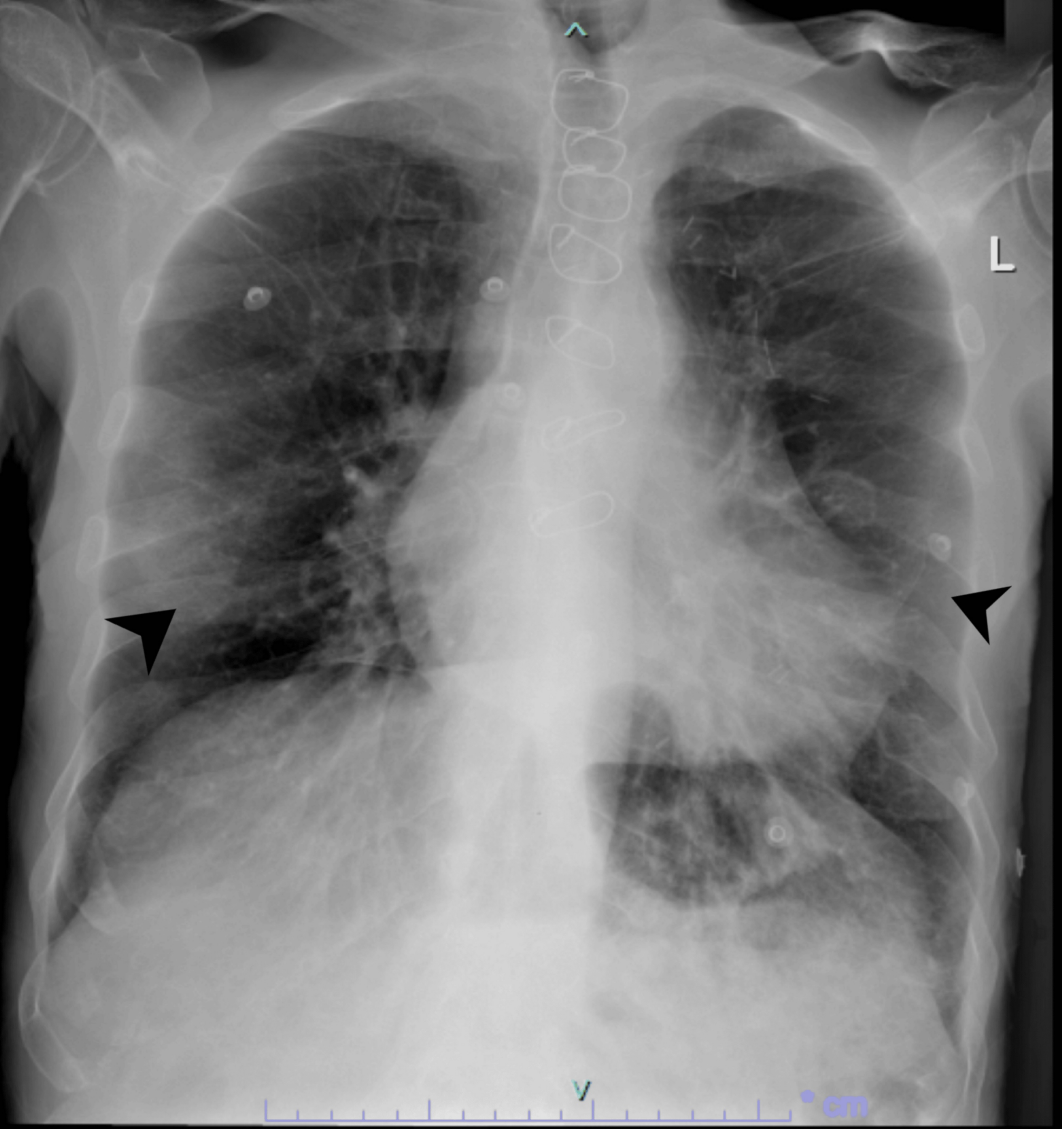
Chest X-ray after two days revealing more obvious bilateral peripheral infiltrates indicated by the arrows, characteristic of Covid-19 pneumonia.
